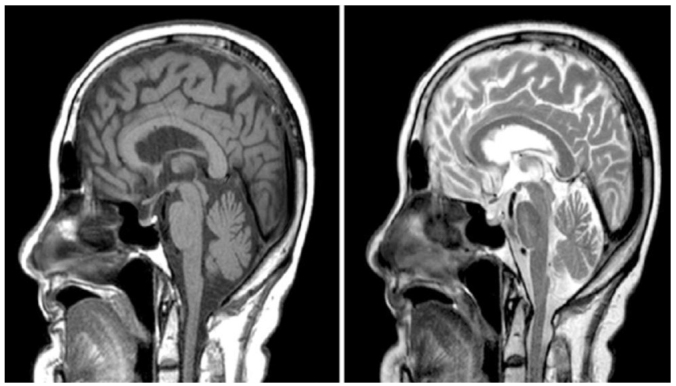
Magnetic resonance imaging (MRI) of the brain showing partial empty sella turcica.
